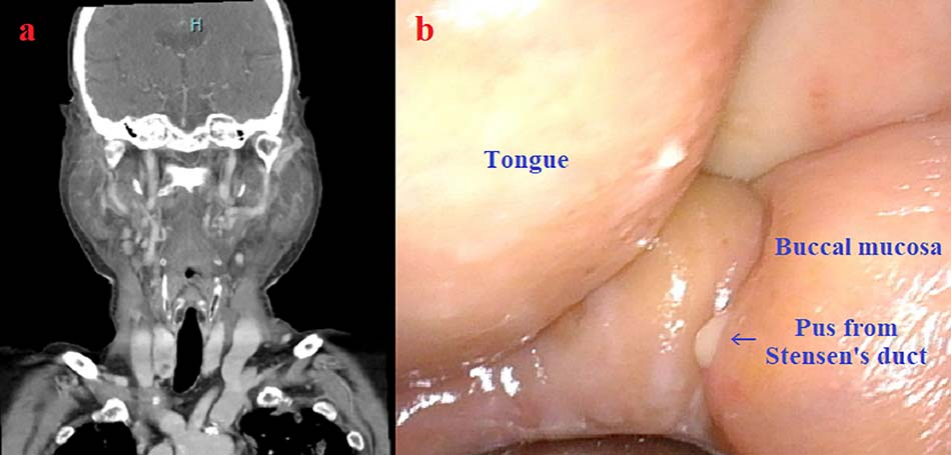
(a) Marked swelling of the bilateral parotid glands shown by computed tomography scan; (b) laryngoscopic view of pus drainage from Stensen’s duct.
